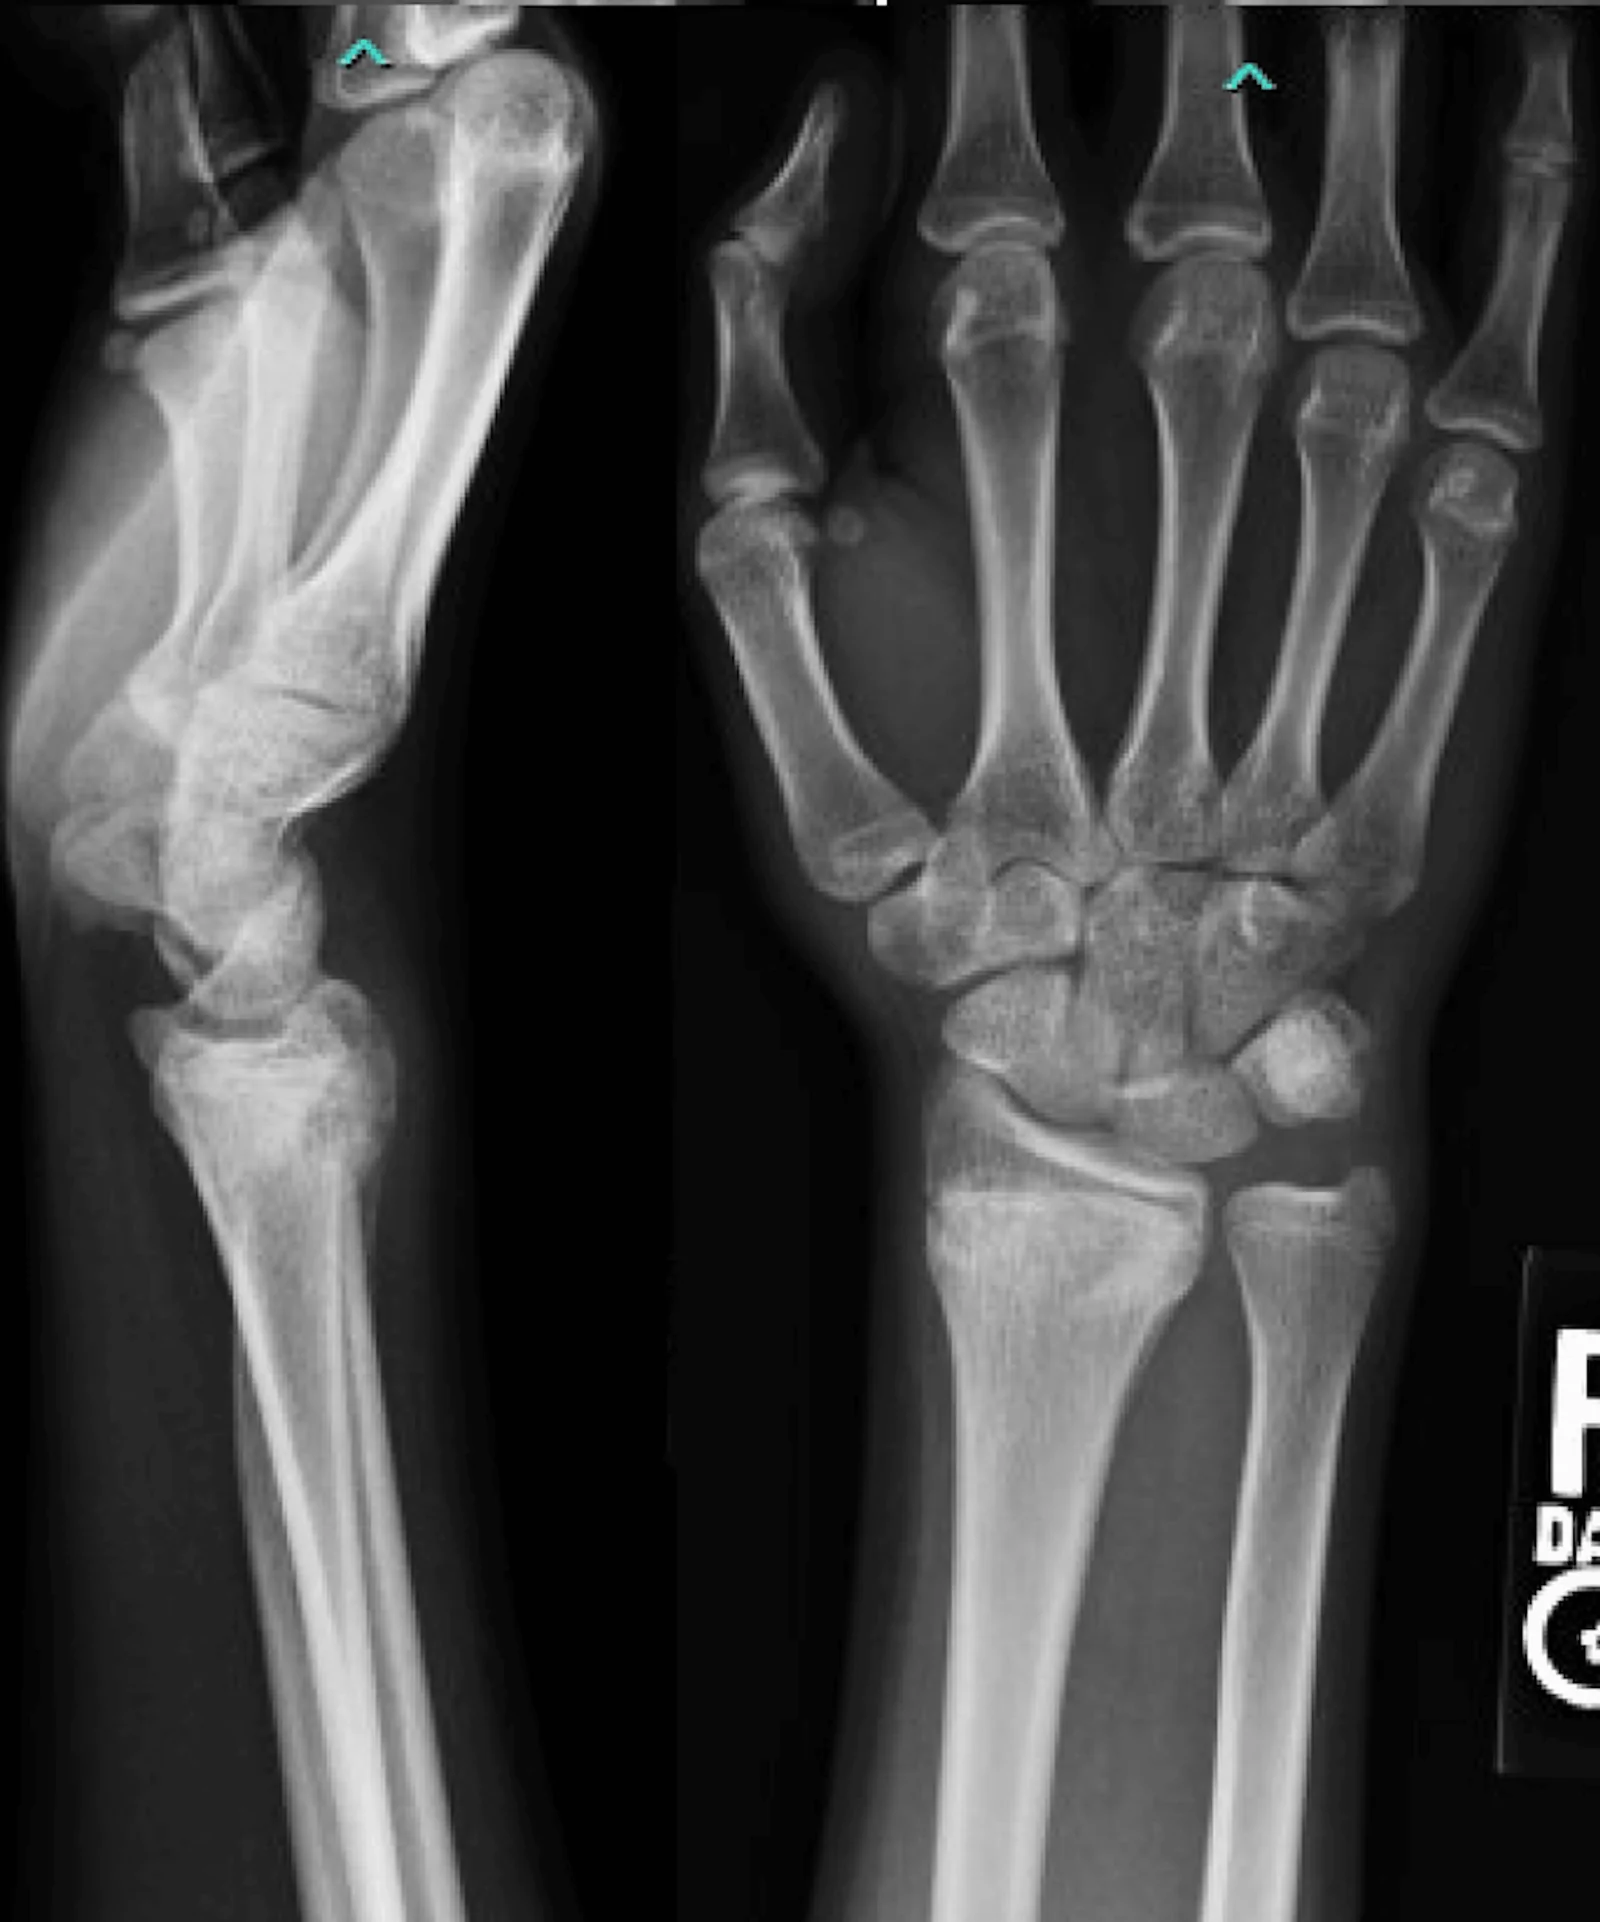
Plain films obtained in clinic at time of evaluation, when EPL rupture was diagnosed. EPL, extensor pollicis longus
